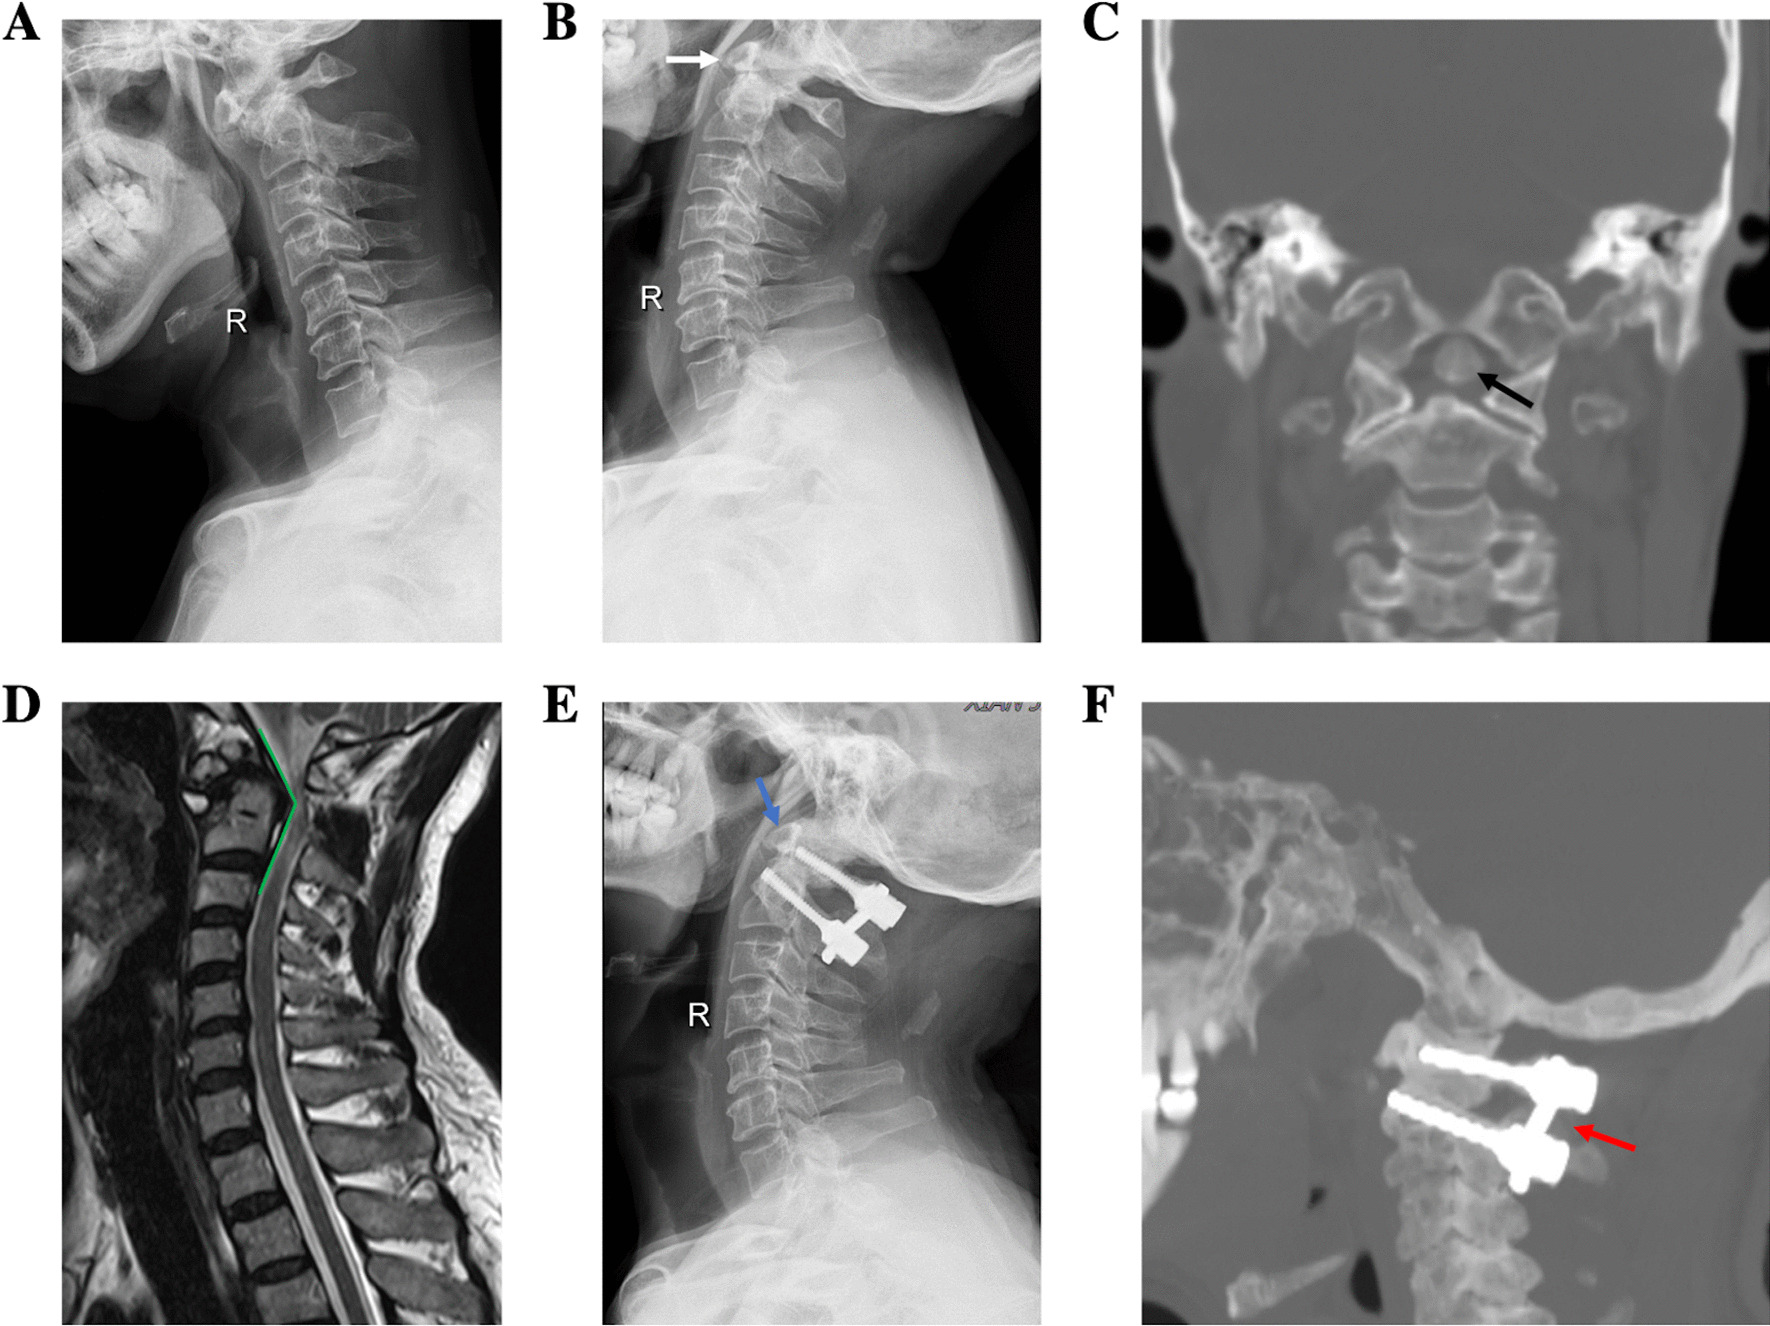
A 50-year-old male patient was admitted for numbness of limbs with walking instability for 6 months, aggravated for 2 months. The ADI was 5.83 mm in preoperative hyperflexion X-ray (a), but the atlantoaxial dislocation could be completely reduced in the hyperextension X-ray (b, white arrow), So it was classified as type I. Computed tomography (CT) coronal reconstruction presented the OO (c, black arrow). The upper cervical spinal cord was compressed by the superior posterior part of axis based on magnetic resonance imaging (MRI), with a cervicomedullary angle of 143° (d). Posterior fixation and fusion were performed. Postoperative X-ray showed atlantoaxial fixation by screw and rod, and anatomical reduction was observed (e, blue arrow). At 6 months after operation, CT reexamination showed posterior bone graft healing (f, red arrow)
